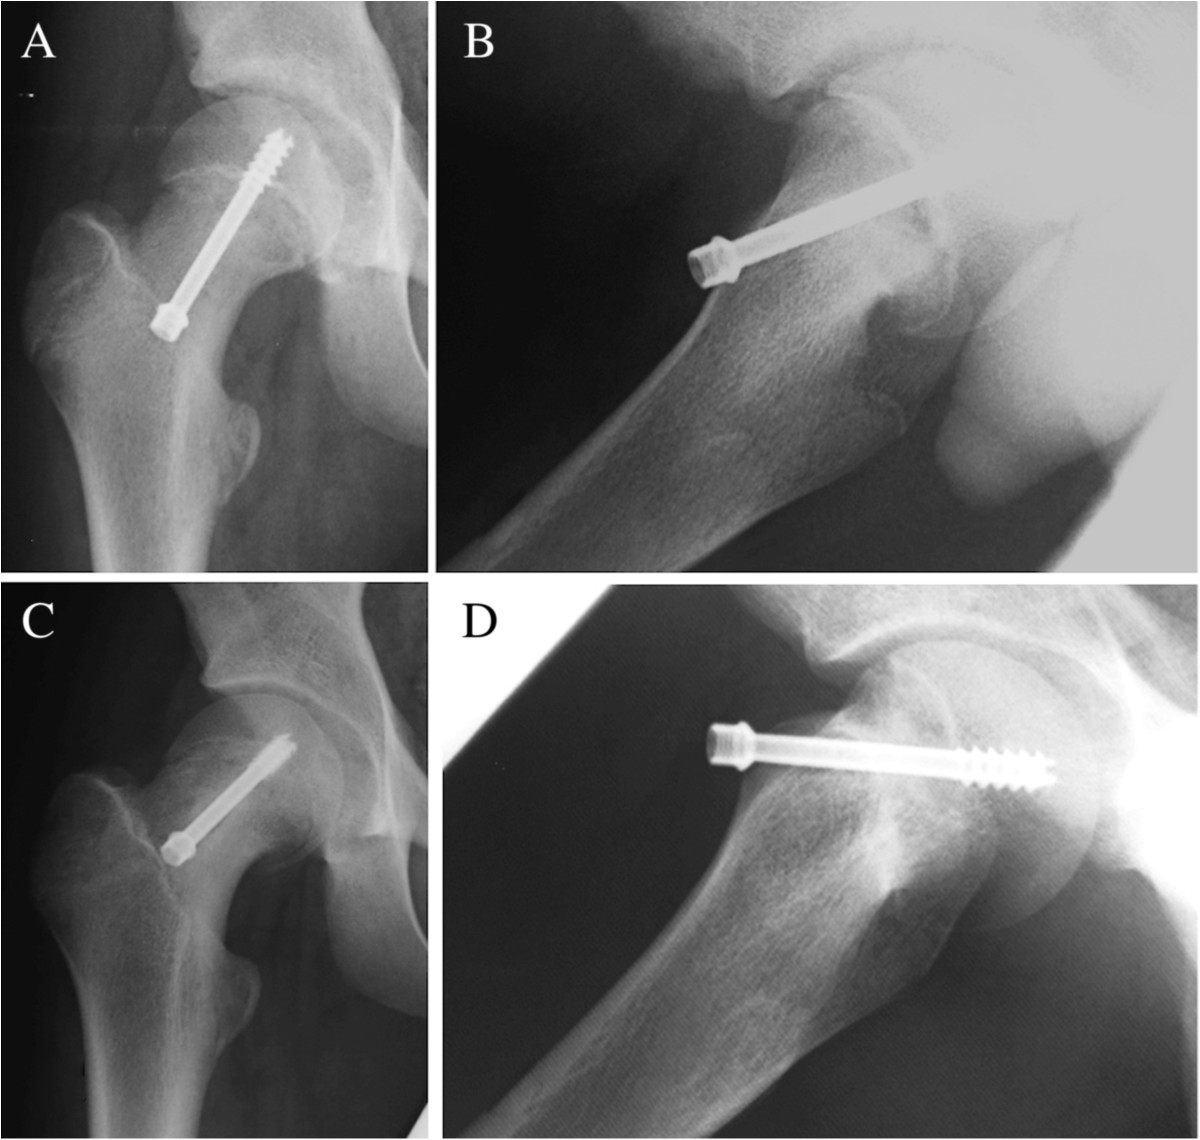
Radiographs of the right hip in a 14-year-old boy with SCFE. Anteroposterior (A) and lateral (B) radiographs immediately after ISF for chronic and stable type hips at 38 degrees of PTA. A single screw was inserted from the medial point to the center of the epiphysis perpendicularly. Anteroposterior (C) and lateral (D) radiographs at physeal closure. Slippage of the epiphysis had progressed to 43 degrees of PTA 17 months after surgery.
